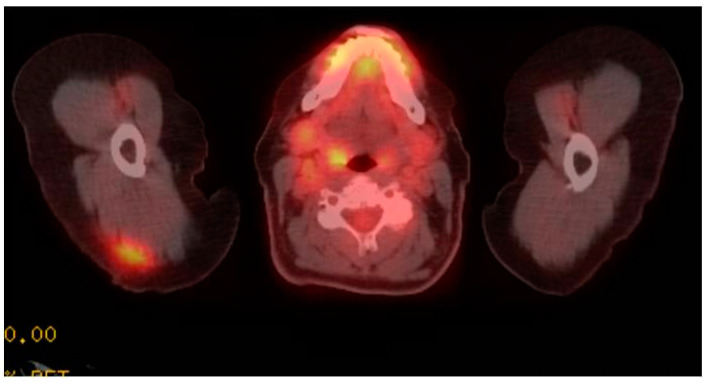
PET/CT imaging shows increased FDG avidity in right deltoid muscle.
